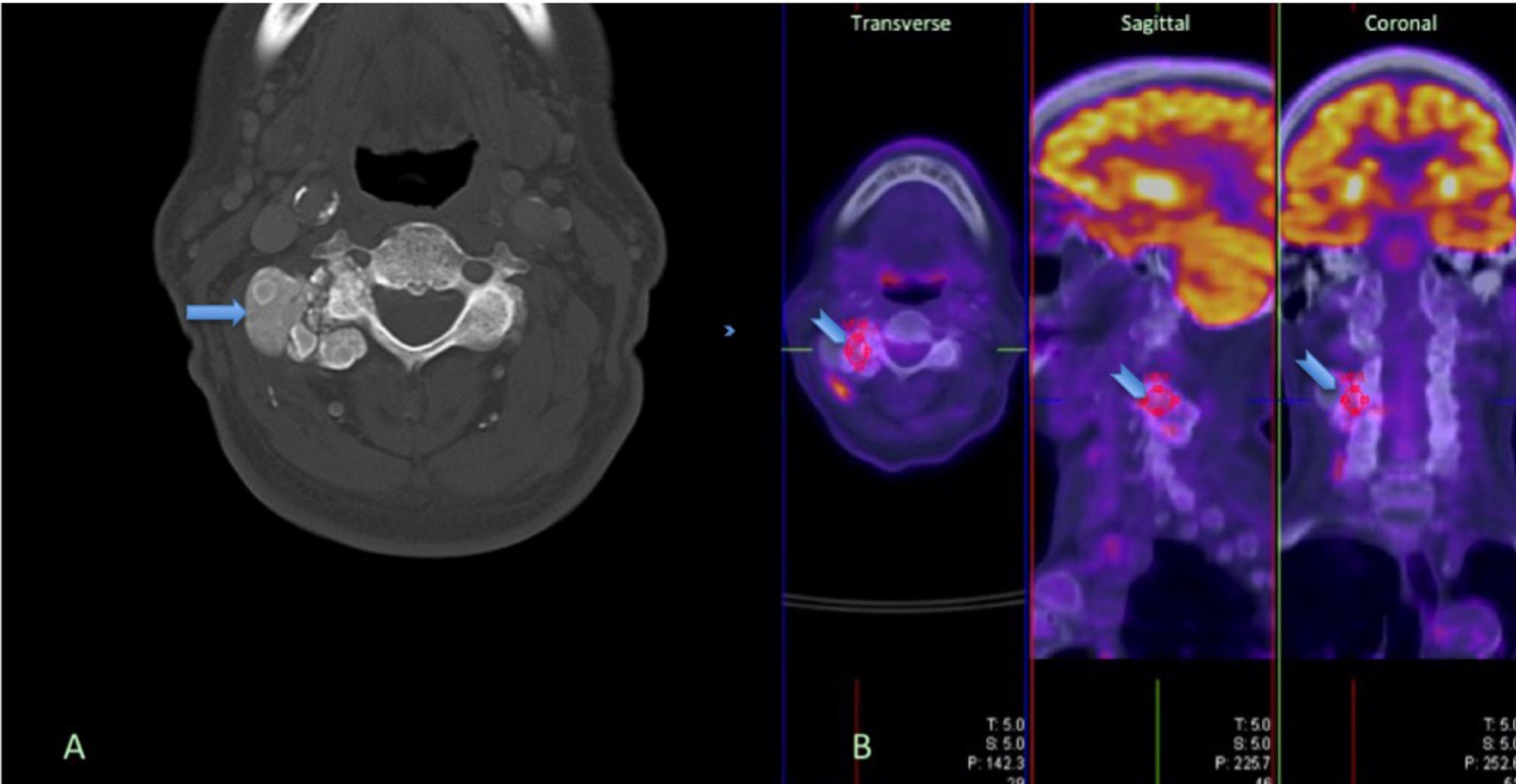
Cervical Tumoral Calcinosis A. Computer tomography (CT) of cervical calcinosis; B. Positron emission tomography-CT of mildly hypermetabolic calcified mass centered at the right C3-C4 facet joint
